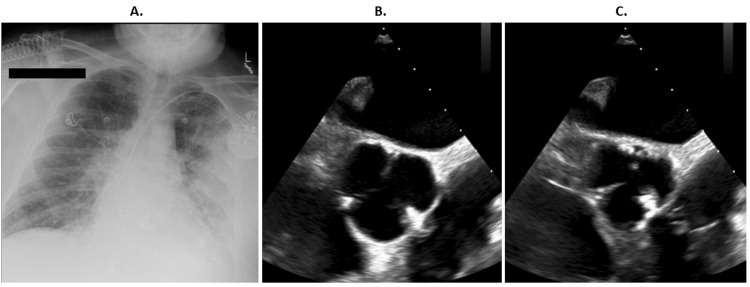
Representative Imaging of Chest X-Ray and Transesophageal Echocardiography A: Chest radiograph demonstrating patchy left-sided airspace opacities consistent with pneumonia. Pulmonary edema was also a possibility, but less likely. B and C: Transesophageal echocardiography images demonstrating a small fibrinous echo density on the left coronary cusp of the aortic valve, which could not exclude infective endocarditis.
